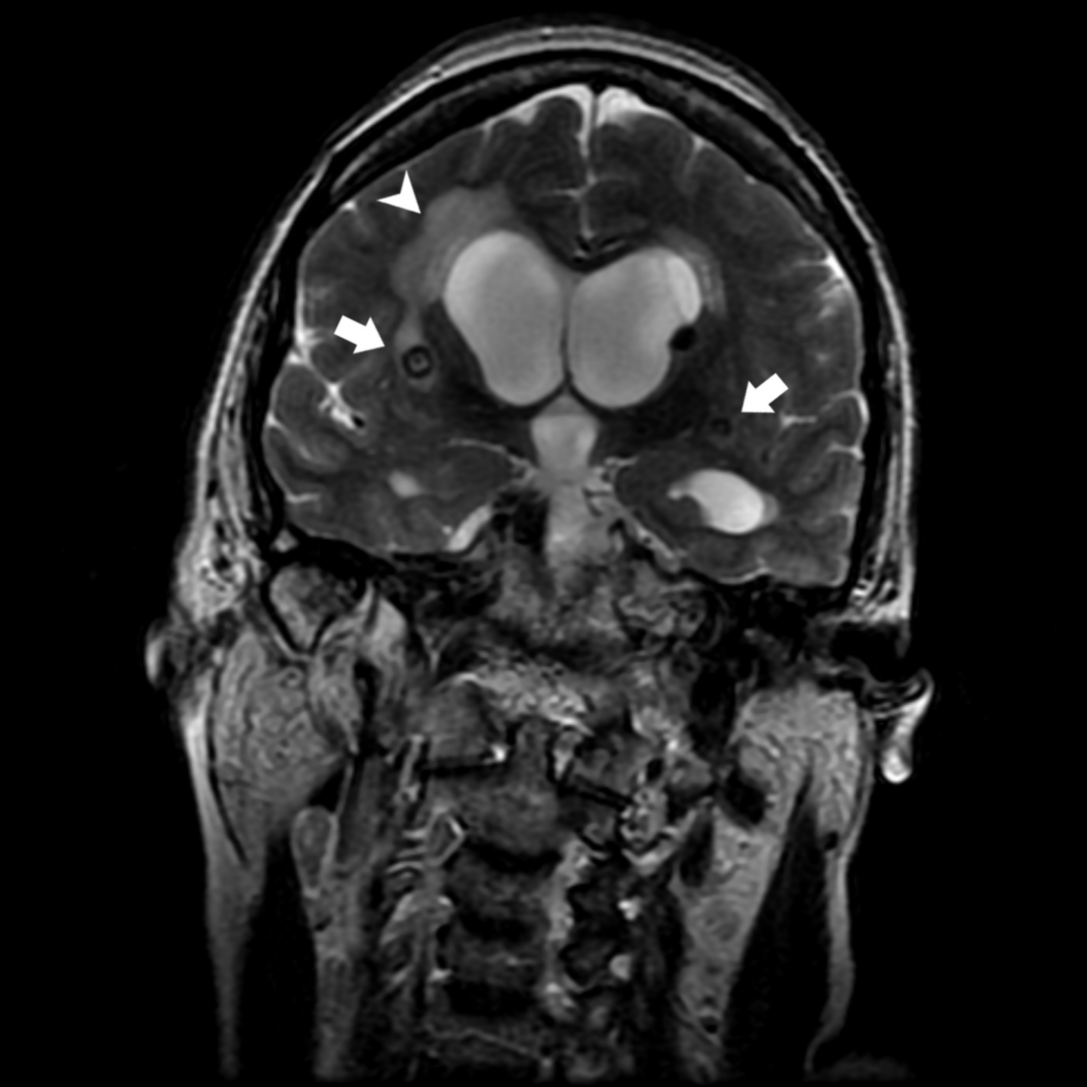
MRI T2-weighted coronal. T2-weighted coronal MRI image showing cysticerci in brain parenchyma (left arrow) and subarachnoid space (right arrow)  and signs of transependymal edema (arrowhead).
